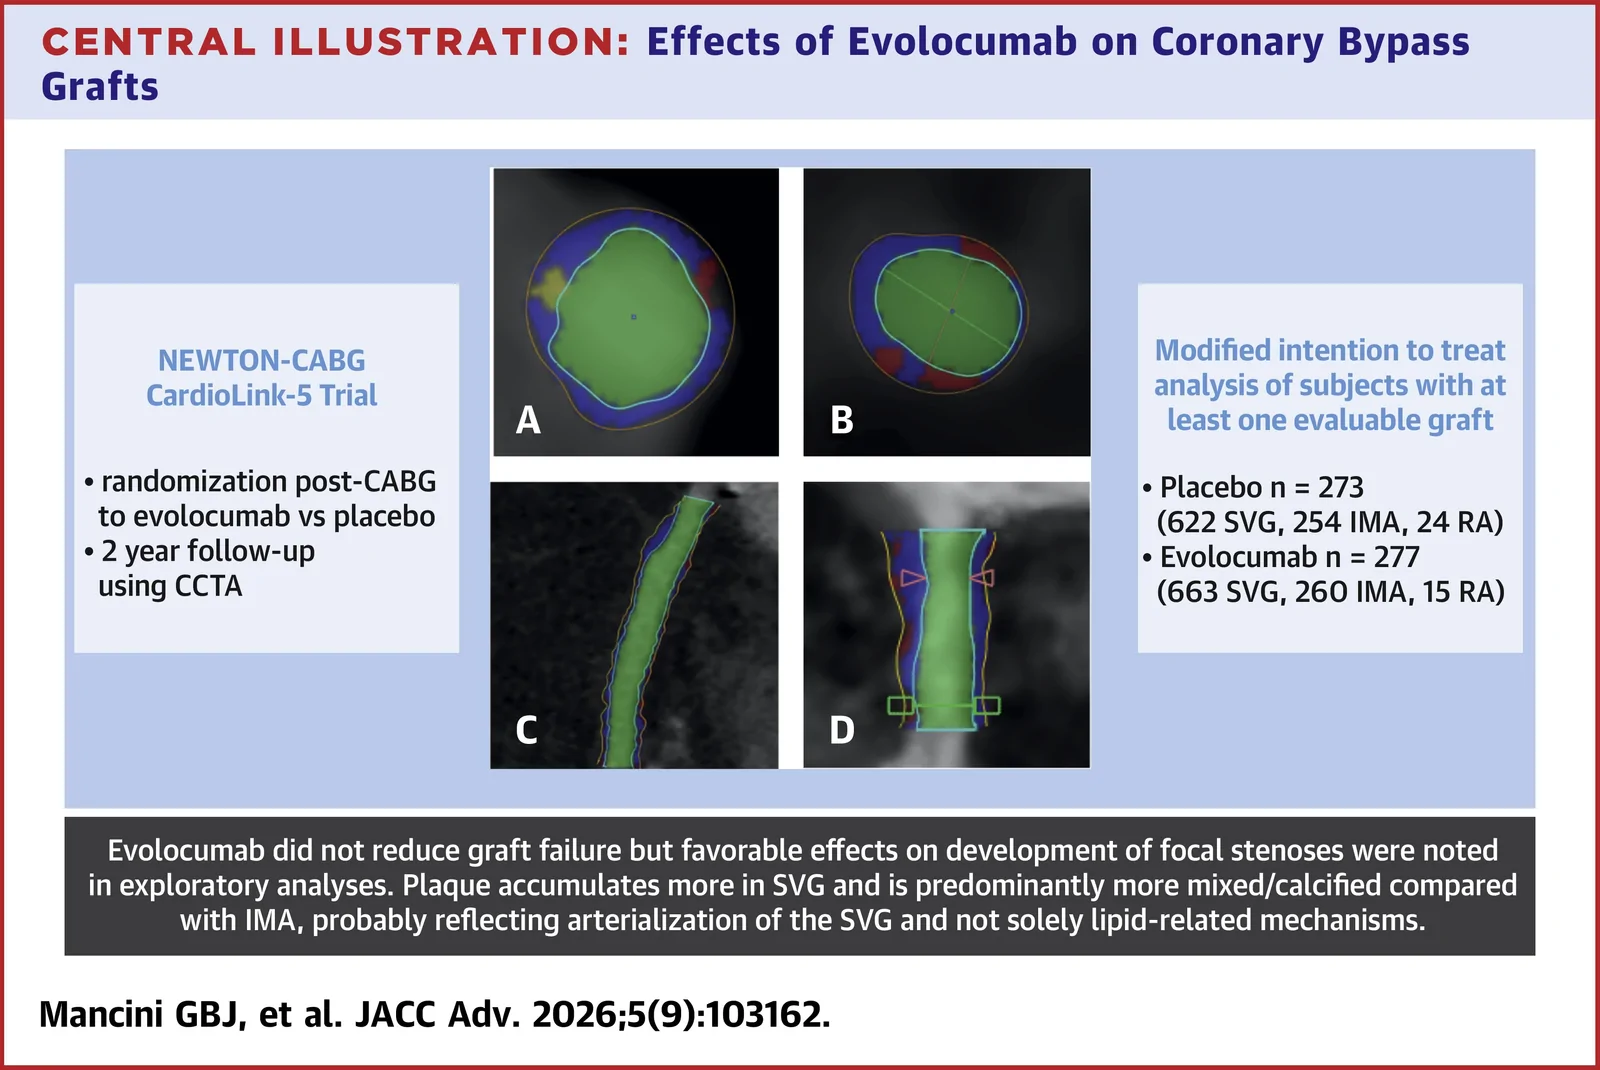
Effects of Evolocumab on Coronary Bypass Grafts Images are color coded: lumen, green; calcification, yellow; mixed plaque, blue; low attenuation plaque, red; external vessel wall, orange. (A) Cross section of a saphenous vein graft demonstrating calcification, mixed plaque, and low attenuation plaque. The low attenuation plaque area is 6.6%. (B) Cross section of a left internal mammary artery graft with low attenuation plaque of 31.4% and no calcium. (C) Longitudinal saphenous vein graft. The wall of the saphenous vein graft demonstrates mixed plaque and areas of low attenuation plaque. No calcium is present. A mild, focal stenosis (25%) is present in the proximal portion. (D) Longitudinal view of a saphenous vein stenosis where the external vessel wall at the site of maximal stenosis (orange outer line at red arrows) is larger than at the reference area (green rectangles) yielding a positive remodeling index of 1.47. CCTA = coronary computed tomography angiography; other abbreviations as in Figures 2 and 3.
